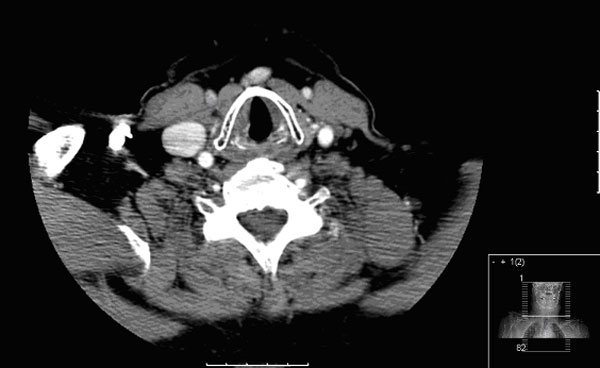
Axial computed tomography through the vocal cords. Computed tomography with contrast from the skull base to the superior mediastinum. No mass was demonstrated along the course of the vagus, or recurrent laryngeal nerve, in the neck, or in the superior mediastinum. Note the abducted left vocal fold in the above slice through the vocal cords.
